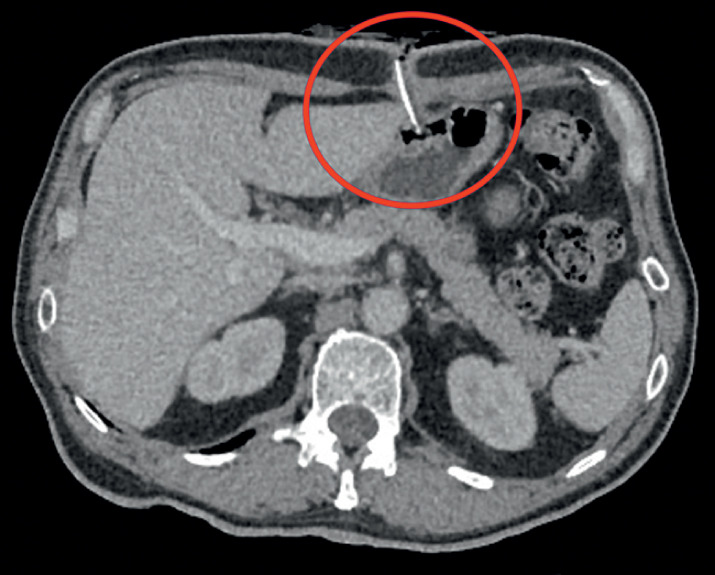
Computed tomography image of the internal bumper of the gastrostomy in the anterior abdominal wall.
